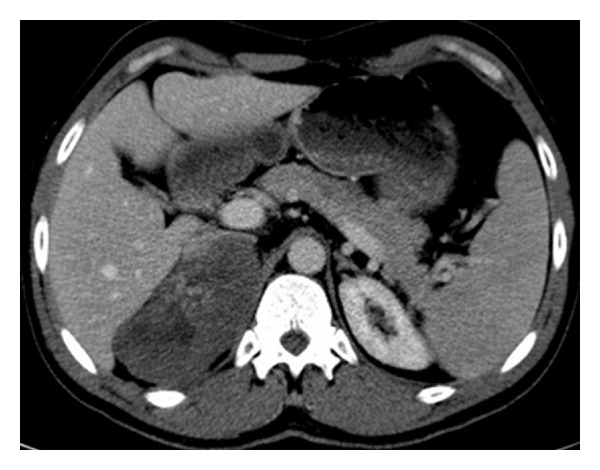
Axial CT scan at the nephrographic phase; there is still patchy central enhancement with nonenhancing peripheral part.
